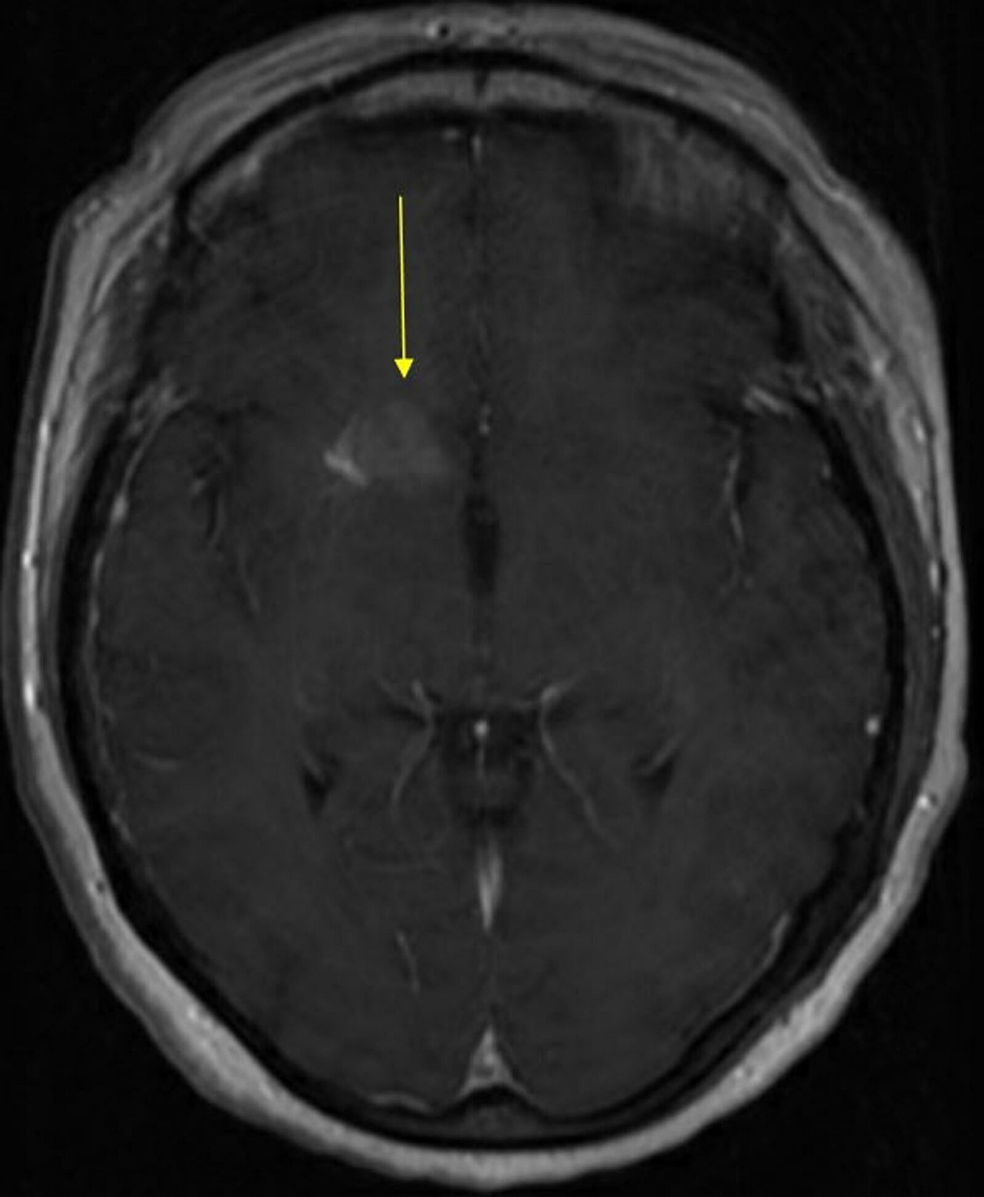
MRI brain with and without contrast, depicting unilateral dense calcification in the right basal ganglia, most likely corresponding to a deep venous anomaly (arrow). MRI: magnetic resonance imaging.
